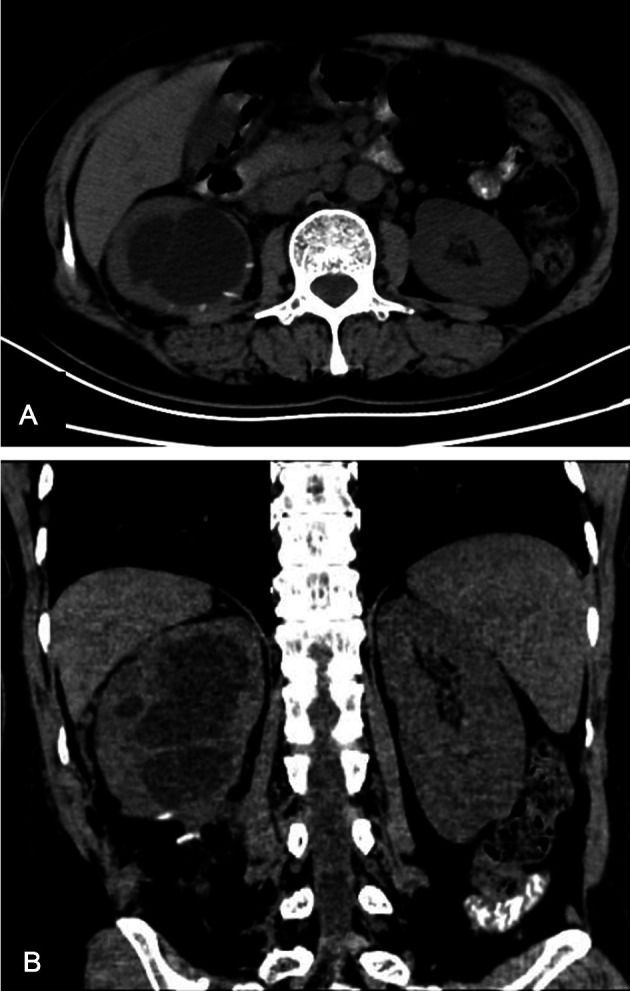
CT scan, which was conducted 3 weeks after drainage tube removal, showing hydronephrosis of the right kidney without perirenal exudation. The lower pole cyst of the right kidney shrank significantly. (A) Sagittal images, (B) coronal image. CT, computed tomography.
